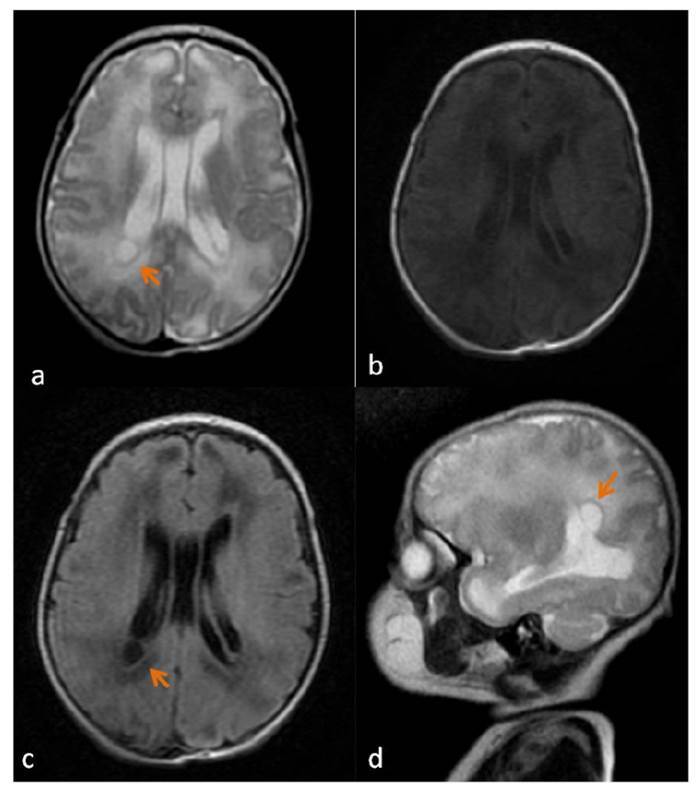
Axial T2-weighted (a), T1-weighted (b), FLAIR (c), and sagittal T2-weighted (d) images show diffuse white matter signal abnormality, a finding indicative of delayed myelination. Subependymal cyst (arrows) at the right posterior periventricular region is also demonstrated.
